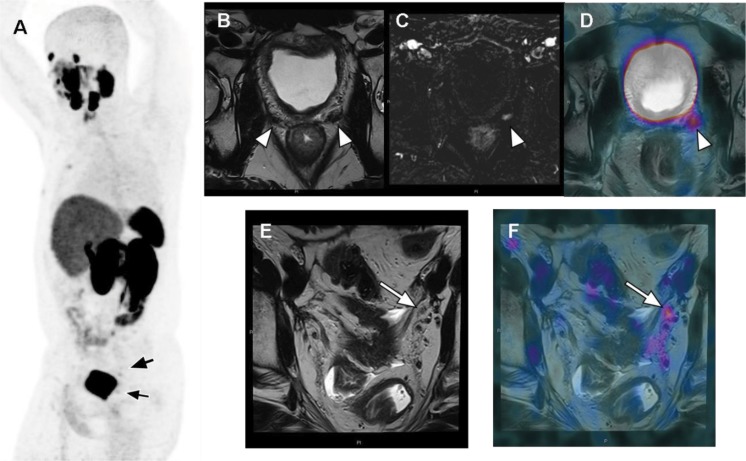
Evaluation of BCR in a 68-year-old patient treated with prostatectomy with a Gleason 7 adenocarcinoma (8 years) and prostate bed radiation (3 years), with a current PSA level of 0.29 ng/ml. ^68^Ga-PSMA-fused PET/MRI MIP images (A) demonstrated 2 pathologic areas of focal uptake in the pelvis (black arrows). Axial T2-weighted imaging (B) showed nonspecific bilateral hypointense tissue in the prostate bed (arrowheads); however, T1-weighted perfusion imaging (C) demonstrated hypervascularity in the left tissue (arrowhead), which also had focal PSMA uptake on fused PET/MR imaging (D), confirming local recurrence. Moreover, a 4-mm left external iliac lymph node (white arrow) was almost not visible on axial T2-weighted imaging (E) but had focal uptake on fused imaging (F), which was very suspicious for pelvic nodal recurrence.
